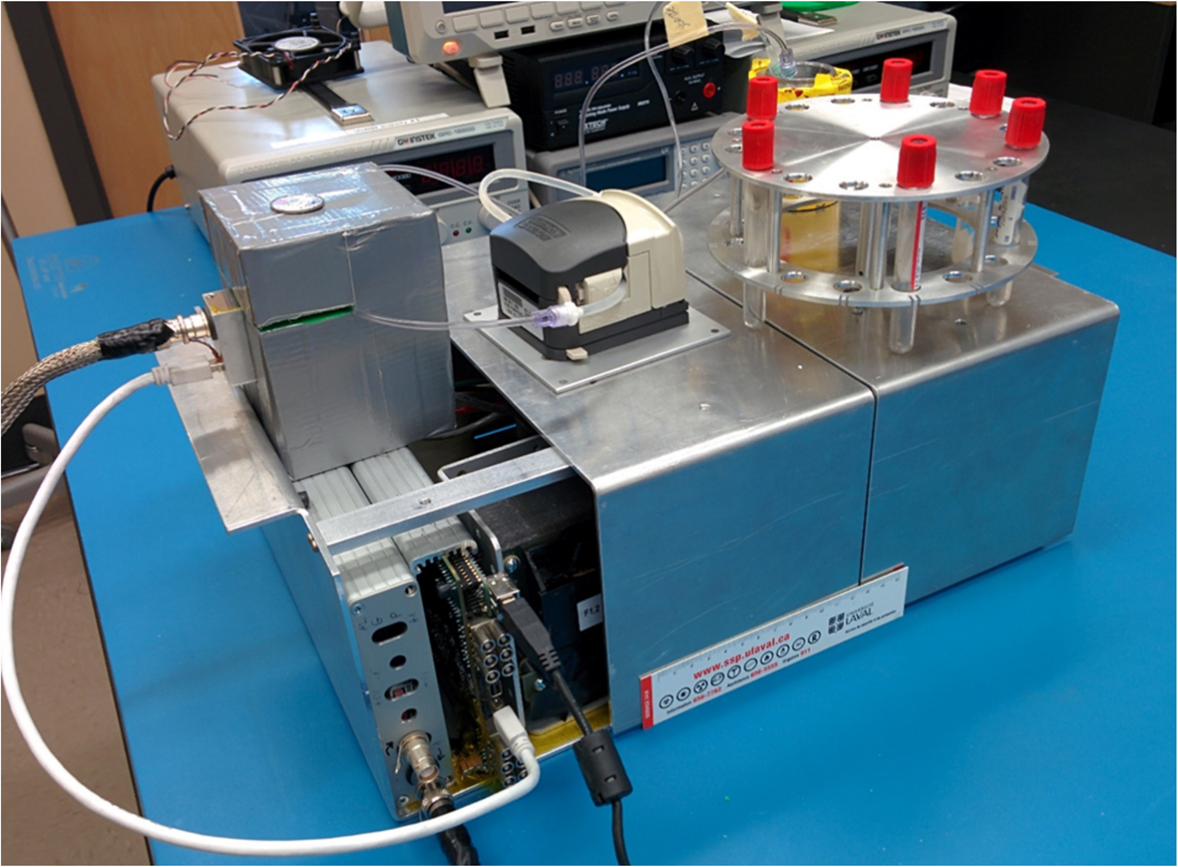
Setup used for calibration with circulating FDG
